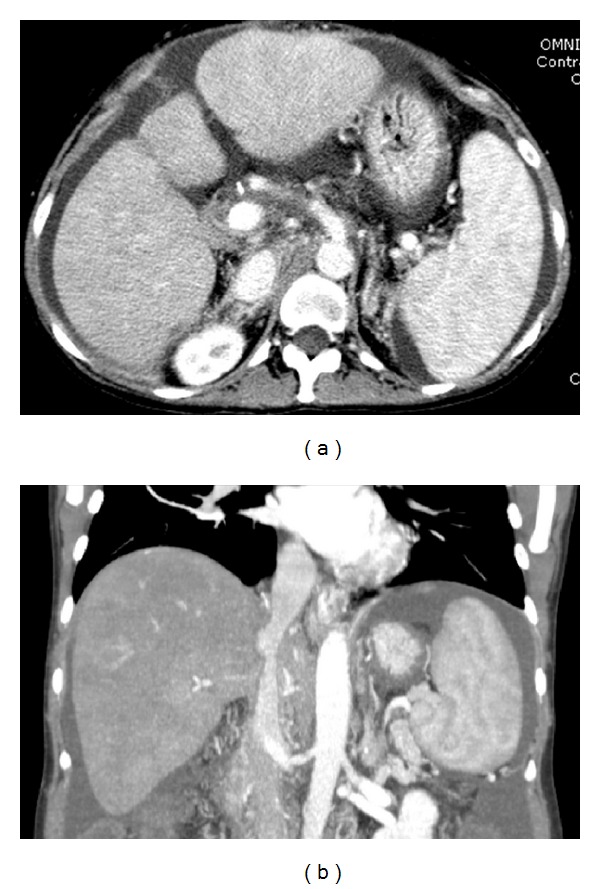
(a) Axial and (b) coronal contrast-enhanced CT of the upper abdomen obtained at admission shows a liver of cirrhotic morphology and nodular contours with mild diffuse heterogeneous enhancement. Splenomegaly, partly visualized ascites, and gastroesophageal varices are also noted. These findings were consistent with cirrhosis and sequela of portal hypertension. Numerous surgical clips about the porta hepatis represent changes of orthotopic liver transplantation.
